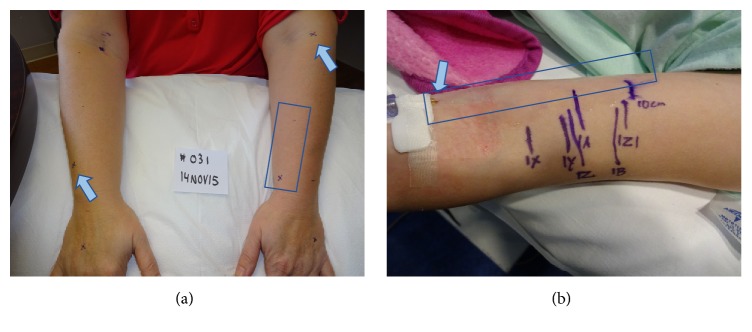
(a) Adult study patient with marked hypothetical insertion sites (arrow) and interrogated segments (box) and (b) pediatric study patient with PIV (arrow) and marked ultrasound findings in 10 cm segment (box).
